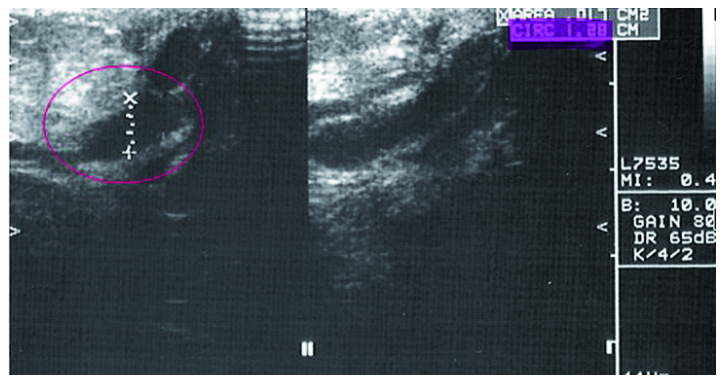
Ultrasonography revealing ductal ectasia on the left and right sides. The pink circle was added by the radiologist to emphasize the duct.
